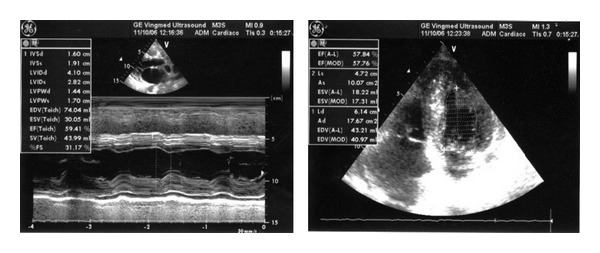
Case 1: echocardiography before bortezomib.
